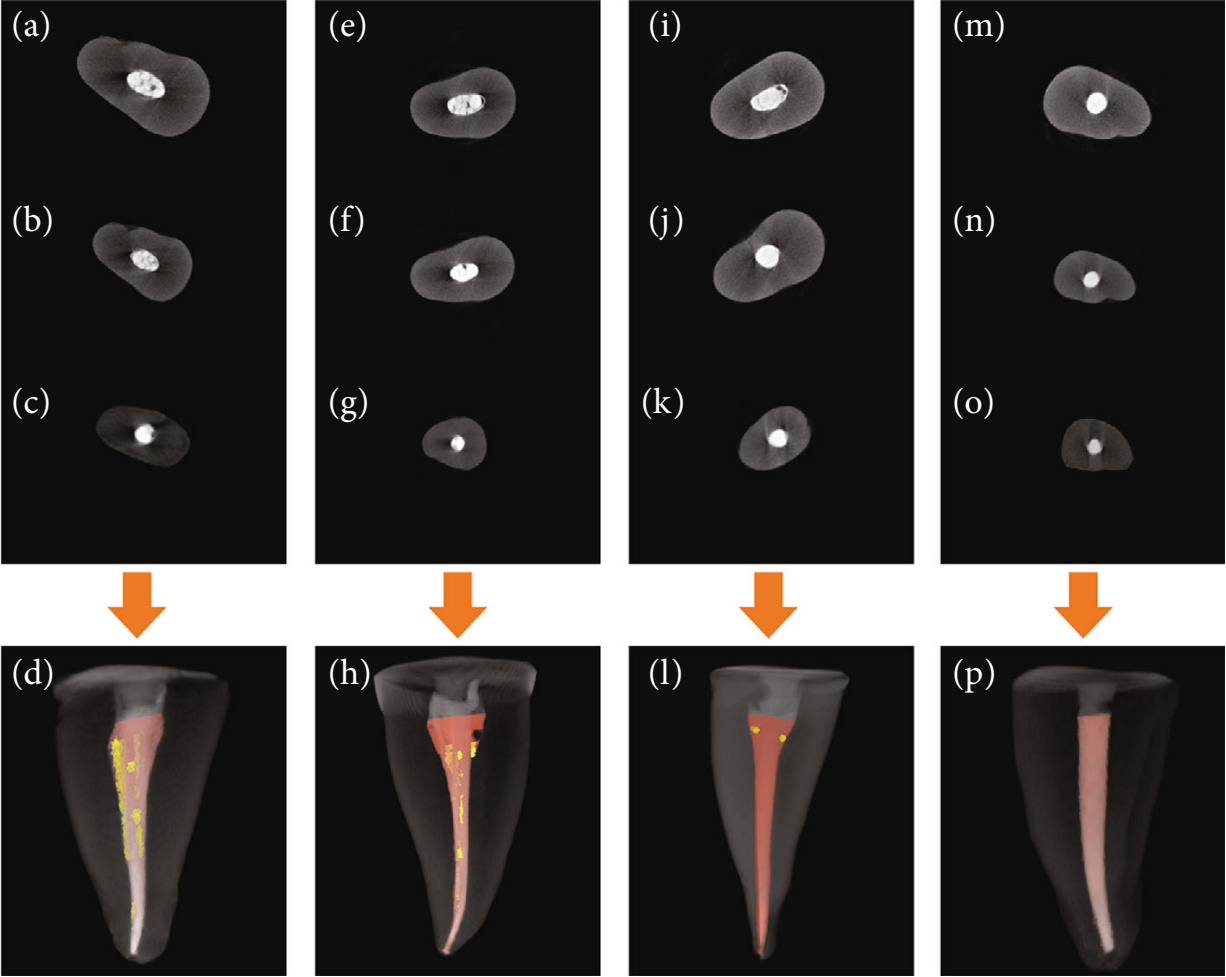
2D axial cross-sections and 3D reconstruction in the root canal filled teeth using different obturation techniques ((a–d) lateral condensation using 0.02 master cone; (e–h) lateral condensation using 0.04 master cone; (i–l) matched single-cone technique; (m–p) matched single cone-mediated ultrasonic activation). In the 3D figures, filling materials (gutta-percha/sealer) are in the orange color, and gaps/voids in the filling materials are in the yellow color.
